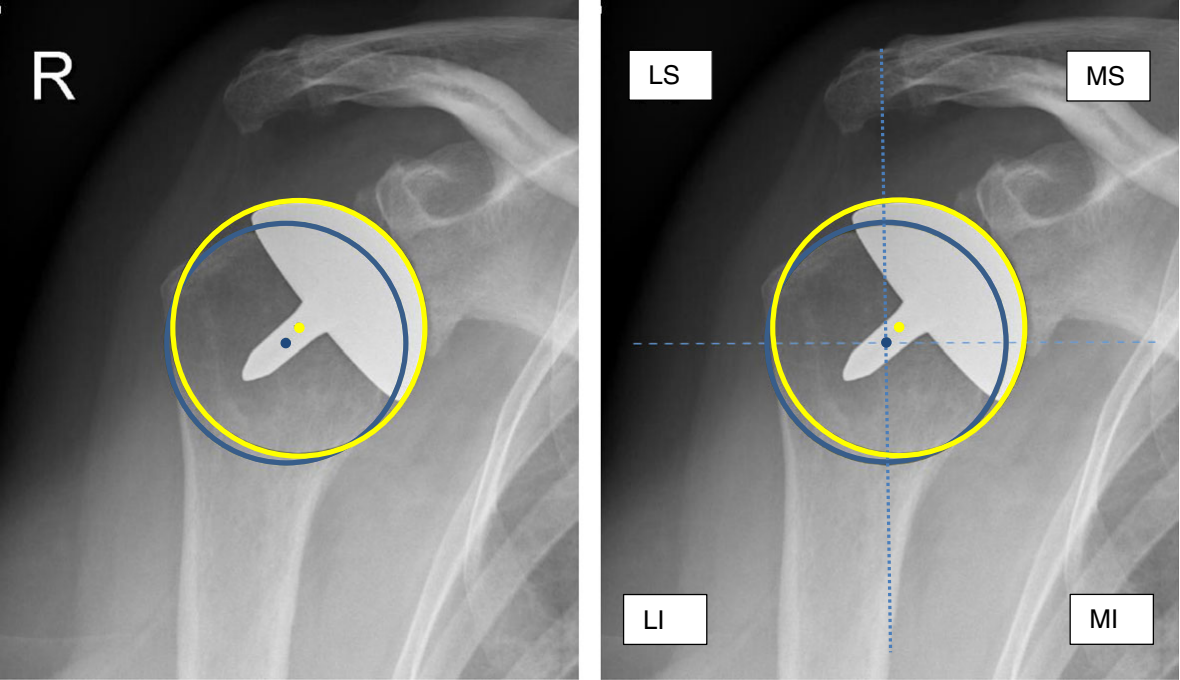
1 Radiograph of a right shoulder. Anatomical circle with center of rotation (blue) and postoperative center of rotation (yellow). The distance in millimeters between the both centers was measured. 2 Radiograph of a right shoulder, demonstrating the anatomical circle (blue) and the implant matched circle (yellow) and the 4 quadrants. LS, lateral superior; MS, medial superior; MI, medial inferior; LI, lateral inferior
